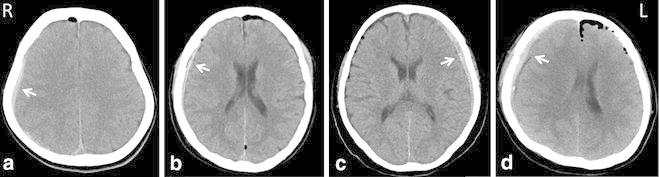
Postoperative computed tomography scans showing unilateral supratentorial subdural hematomas in four patients (*arrows*). a Case 1, b case 2, c case 3, d case 4
